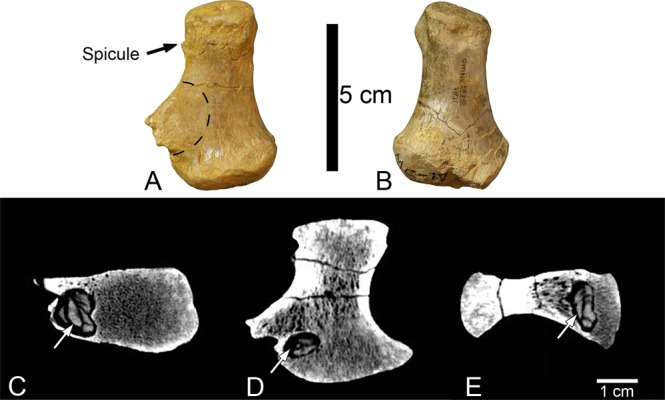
OMNH 58340 left and right metacarpal IV. (A) Pathological left metacarpal IV dorsal view, dashed line shows the location of a disruption in the periosteal surface texture, and the black arrow points to a 3 mm long projection on the proximal margin of the collateral ligament pit. (B) Right metacarpal IV dorsal view. (C–E) Computed tomography scans of left metacarpal IV showing the internal morphology consistent with a type of subacute pyogenic osteomyelitis called a Brodie abscess. (C) Proximal view (D). Dorsal view (E). Lateral view. Arrow indicates the presence of an irregular sequestrum within the abscess consistent with the morphology of a Brodie abscess in an early stage of its development.
